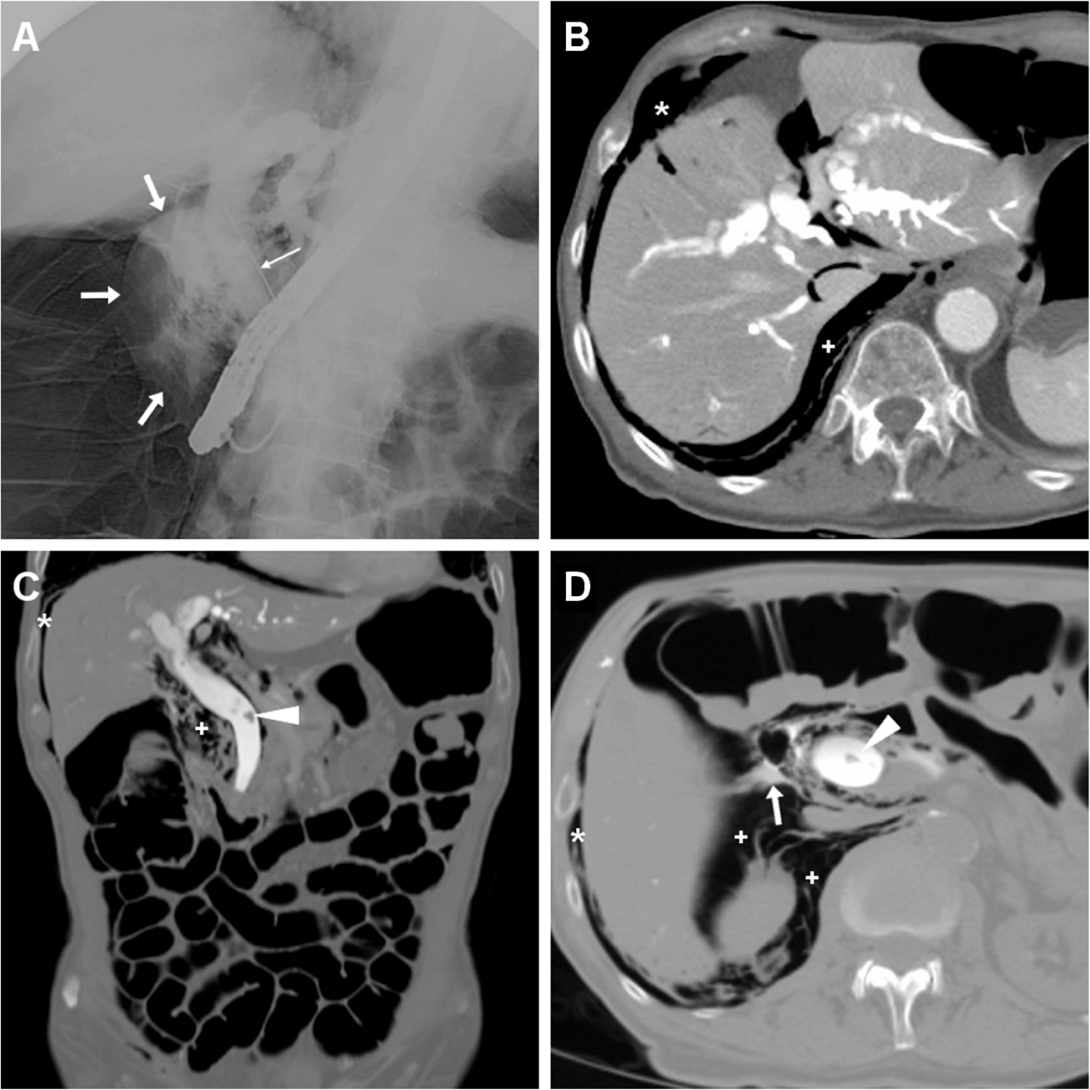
An elderly 80-year-old male with multiple comorbidities underwent endoscopic treatment of choledocholithiasis, including pre-cut sphincterotomy of the Vaterian papilla and stone retrieval using a basket device. During ERCP, fluoroscopy (a) showed opacification of dilated intrahepatic and common bile ducts and of a sizeable extraluminal CM collection (*thin arrows*) from lateral duodenal wall perforation (type I according to the Stapfer classification system). A few hours later CT (b–d) showed diffuse gaseous bowel distension from insufflation during endoscopy, presence of posterior pneumomediastinum, perihepatic and right parietocolic pneumoperitoneum (*), and extensive retroperitoneal emphysema in the right perirenal, posterior and anterior pararenal spaces (+). Note persistently opacified intrahepatic and common bile ducts with residual lithiasis fragments (*arrowheads*), persistently extravasated CM (*arrow* in d). Imaging findings and worsening clinical conditions with peritonitis dictated urgent laparotomy, which confirmed intra-abdominal free air, and included opening and toilette of common bile duct and positioning of a Kehr T-tube. The patient finally recovered after a prolonged hospital stay
